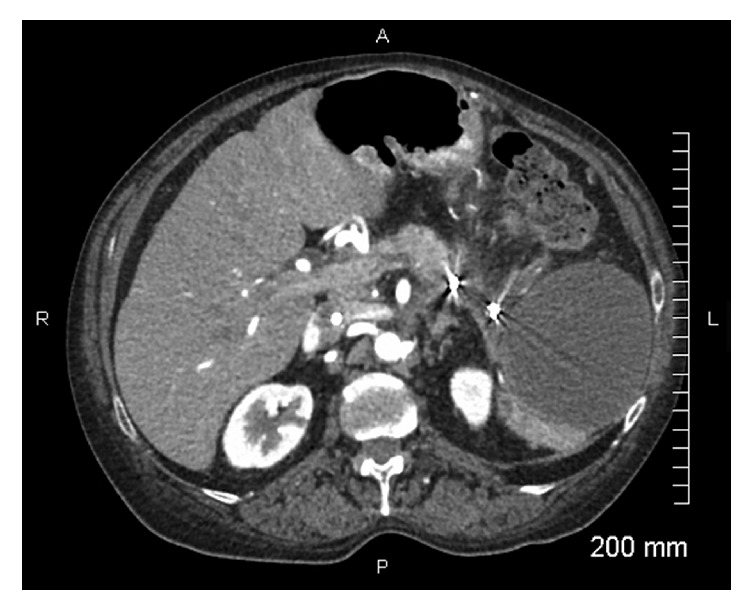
CT image: infarcted spleen.
